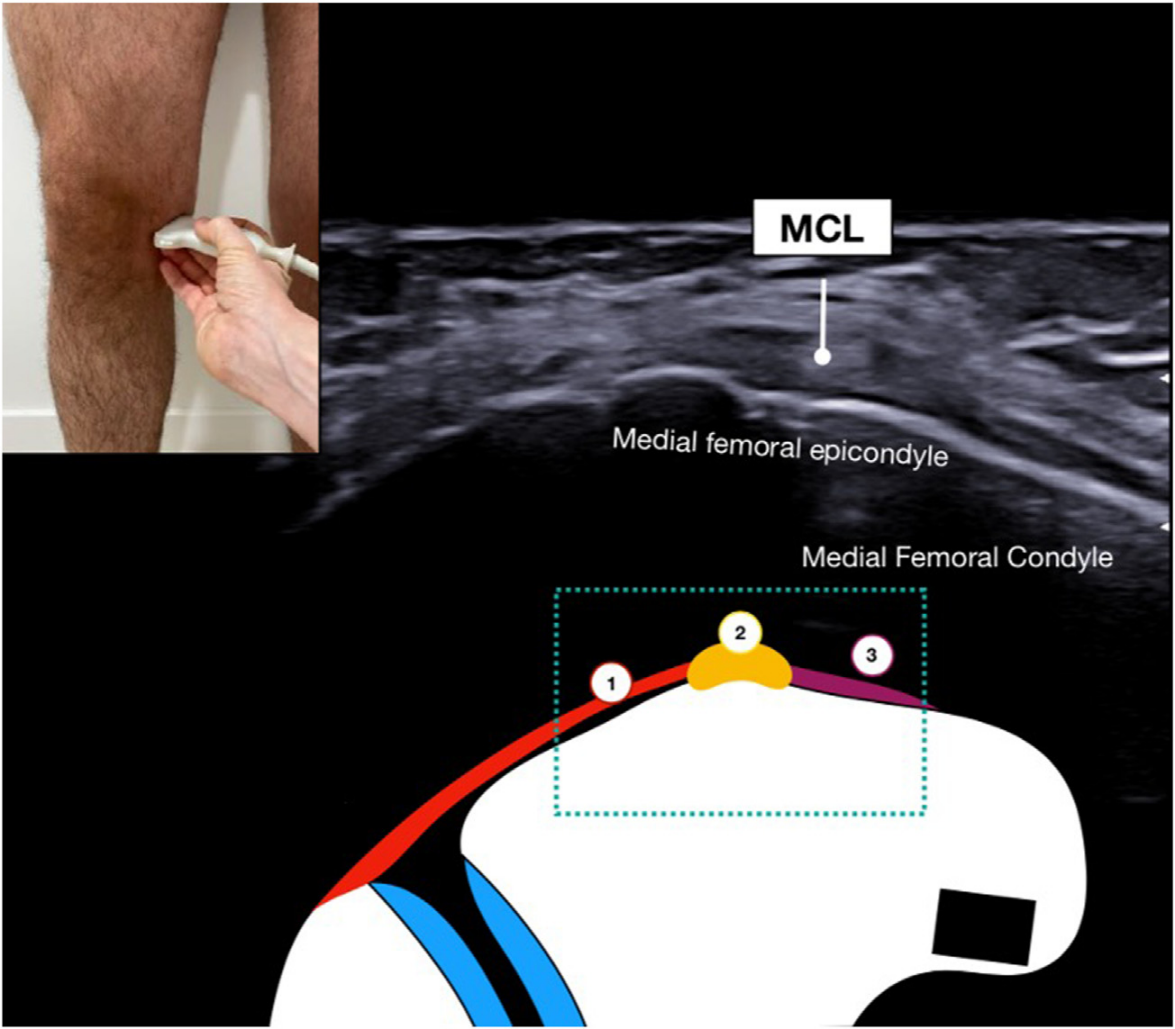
When measuring medial ME from an ultrasound image, the first step is identifying the MCL. Begin by placing the probe over the medial femoral epicondyle in the short-axis plane. The MPFL can be found anterior to the MCL, whereas the POL can be found posterior to the MCL. (MCL, medial collateral ligament; ME, meniscal extrusion; MPFL, medial patellofemoral ligament; POL, posterior oblique ligament.)
